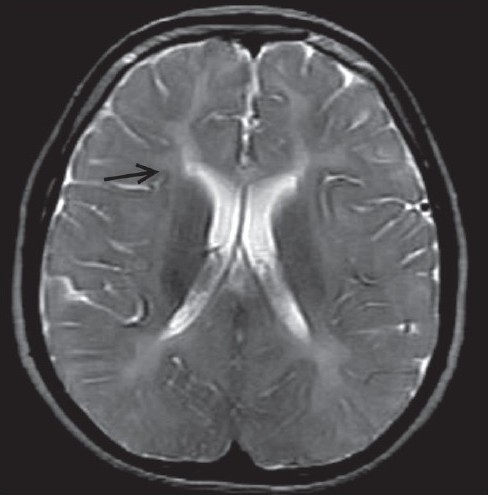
T2W axial magnetic resonance image in a 10-year-old child showing bilateral symmetrical subtle hyperintense lesions (arrow) in the white matter
